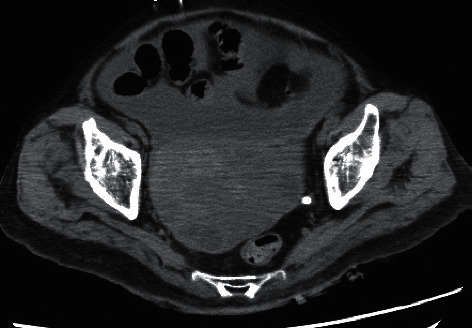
Plain computed tomography (CT) on admission showing ascites accumulation and fluid-containing uterine fibroids.
